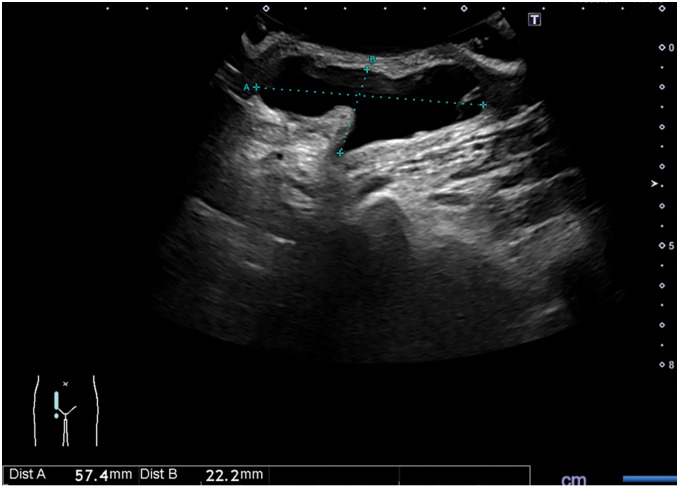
Ultrasonography of the right groin. A cystic mass with low-level internal echoes and no blood flow on color Doppler was observed, measuring 57.4 mm in maximum diameter.
